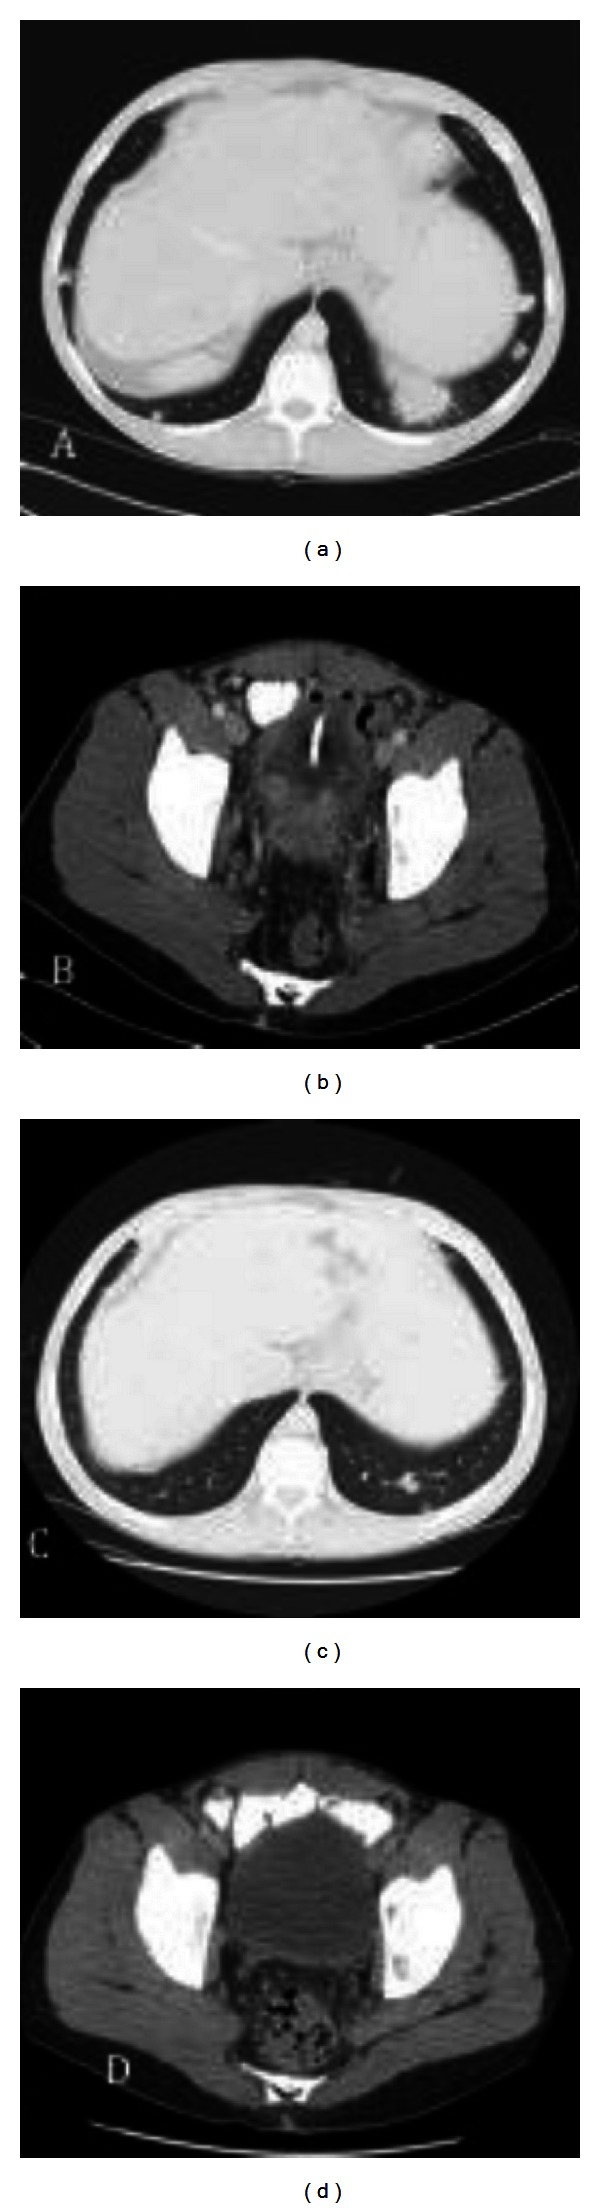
CT scans showing dominant pulmonary nodule in left lower lobe and bladder thickening, pre-chemotherapy (panels A and B), and two months after completion of chemotherapy (panels C and D). These scans demonstrate the stability of the pulmonary nodules, and resolution of the pelvic mass and lymphadenopathy and his subcutaneous nodules.
